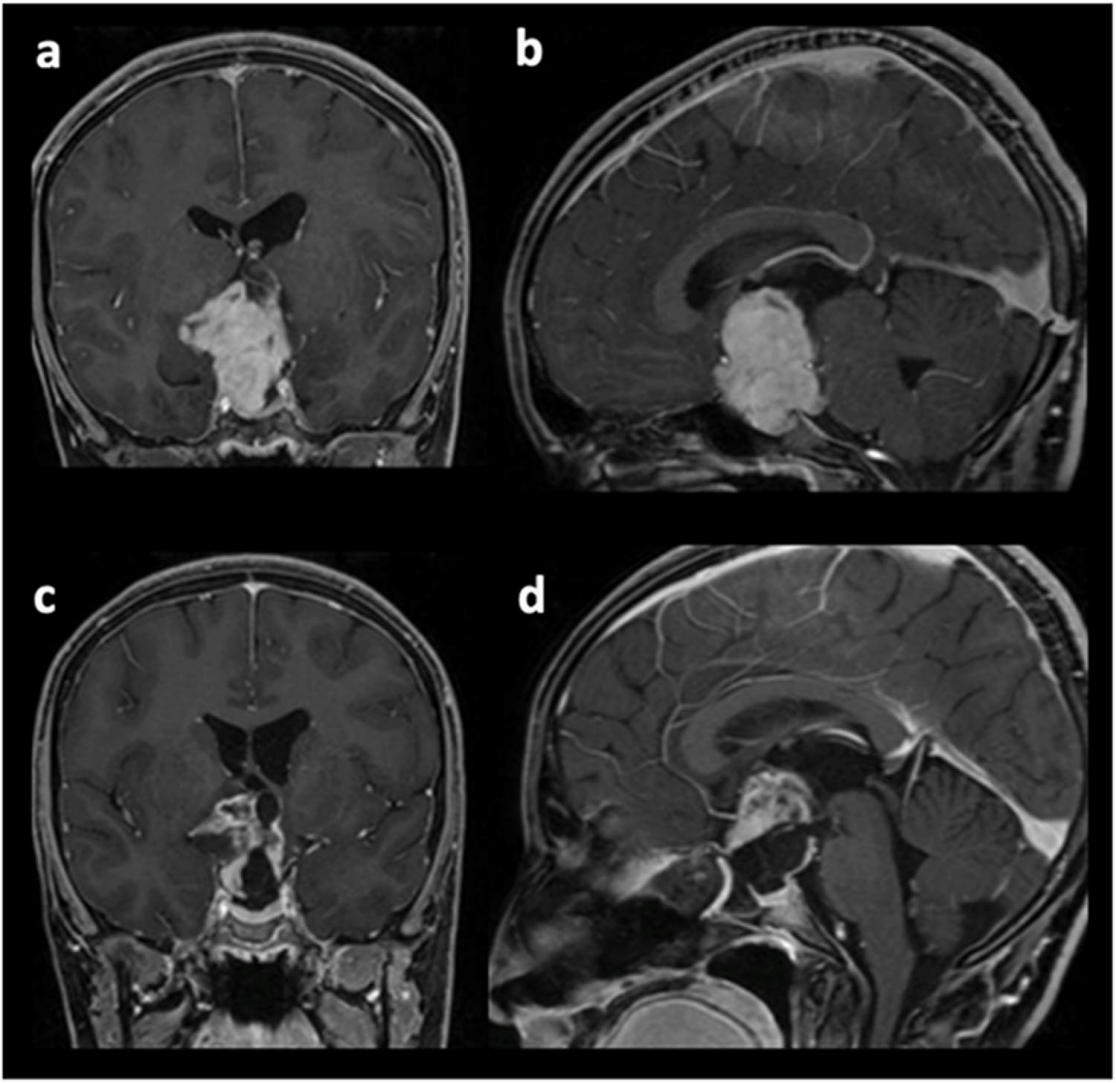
Pilocytic astrocytoma of the optic pathways in a 15-year-old female: a, b coronal and sagittal preoperative T1-weighted contrast-enhanced MRI; c, d coronal and sagittal postoperative imaging, showing planned partial resection and optic pathways decompression
